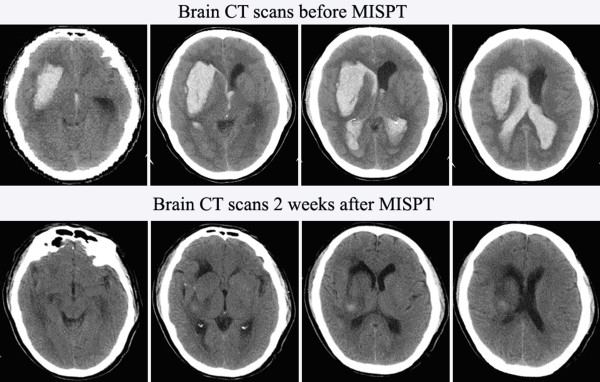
CT scans of one patient in a coma(GCS score 6)with a huge haematoma(> 70 mL) before MISPT(upper), and CT scans of the same patient in consciousness (GCS score 14) 2 weeks after completed MISPT with urokinase (lower). All scans demonstrate a series size of hematoma on axial imaging.
